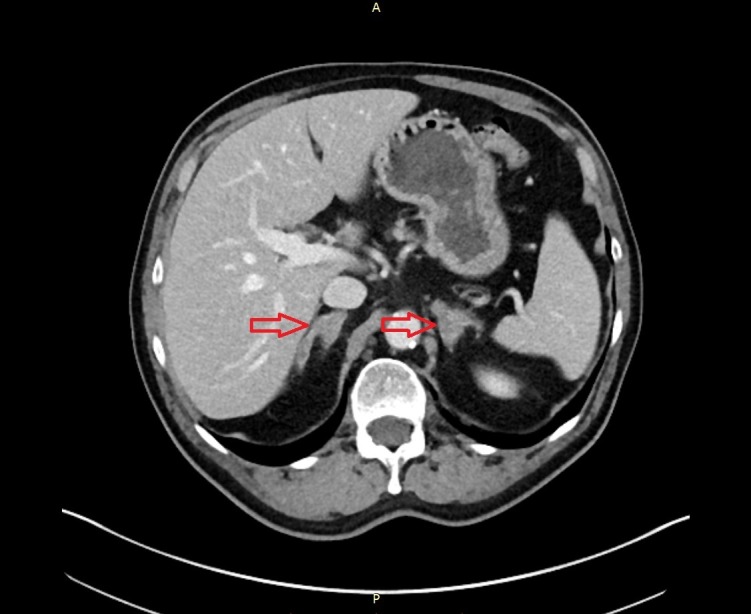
CT scan. The image shows both adrenal glands distended, probably caused by metastases. CT: computed tomography
